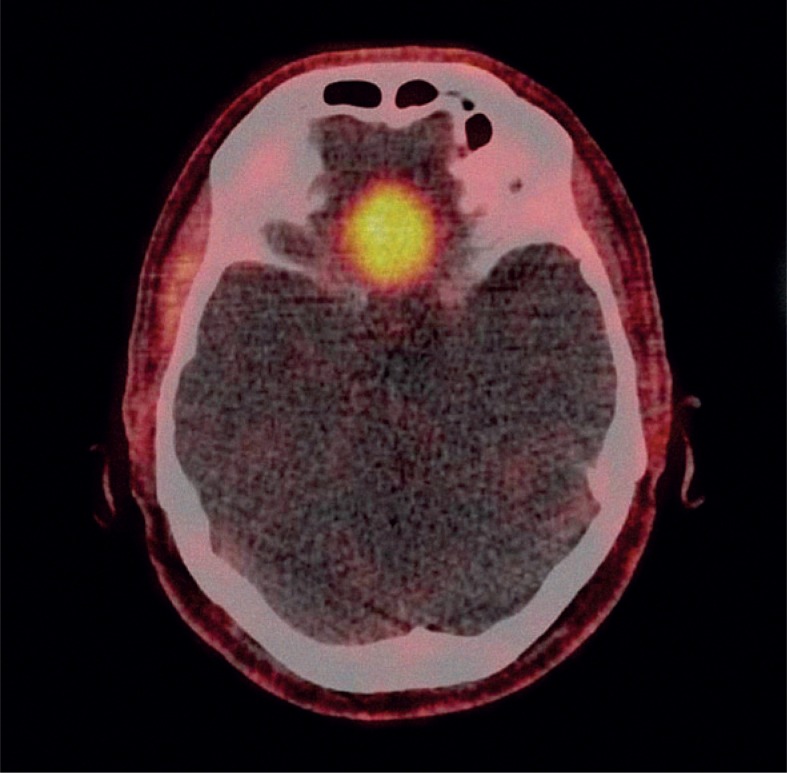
Positron emission tomography-computed tomography axial slice: Meningioma in the anterior cranial fossa that incidentally took-up ^18^F-fluorocholine (SUVmax 3.7).
